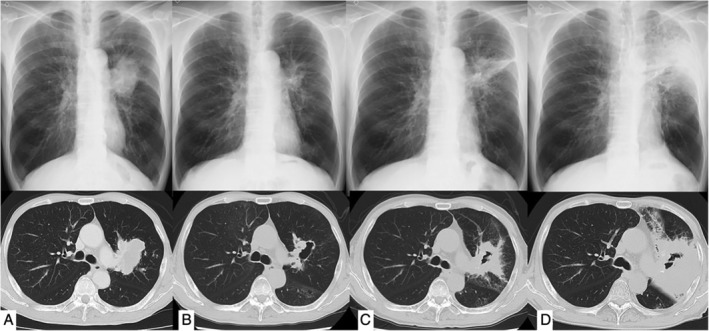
(A) Computed tomography and X‐ray images before treatment showing lung tumour in left lung. (B) After chemoradiotherapy, primary lesion is smaller, forming a cavity. (C) On admission, note the thickening of the cavity wall and consolidation around the cavity. (D) Images on the seventh day after admission showing worsening of the findings despite the initiation of micafungin treatment.
